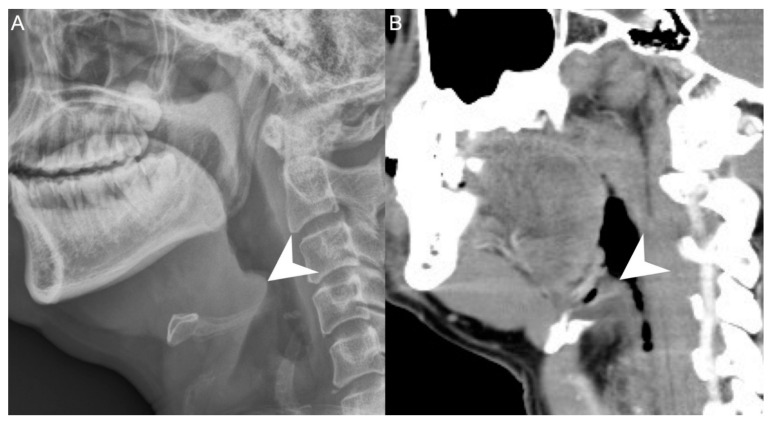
A patient with concurrent acute epiglottitis and deep neck infection as seen from a (A) neck lateral view and (B) sagittal view on CT. Arrowhead: swelling of the epiglottis. 300 × 300 DPI.
